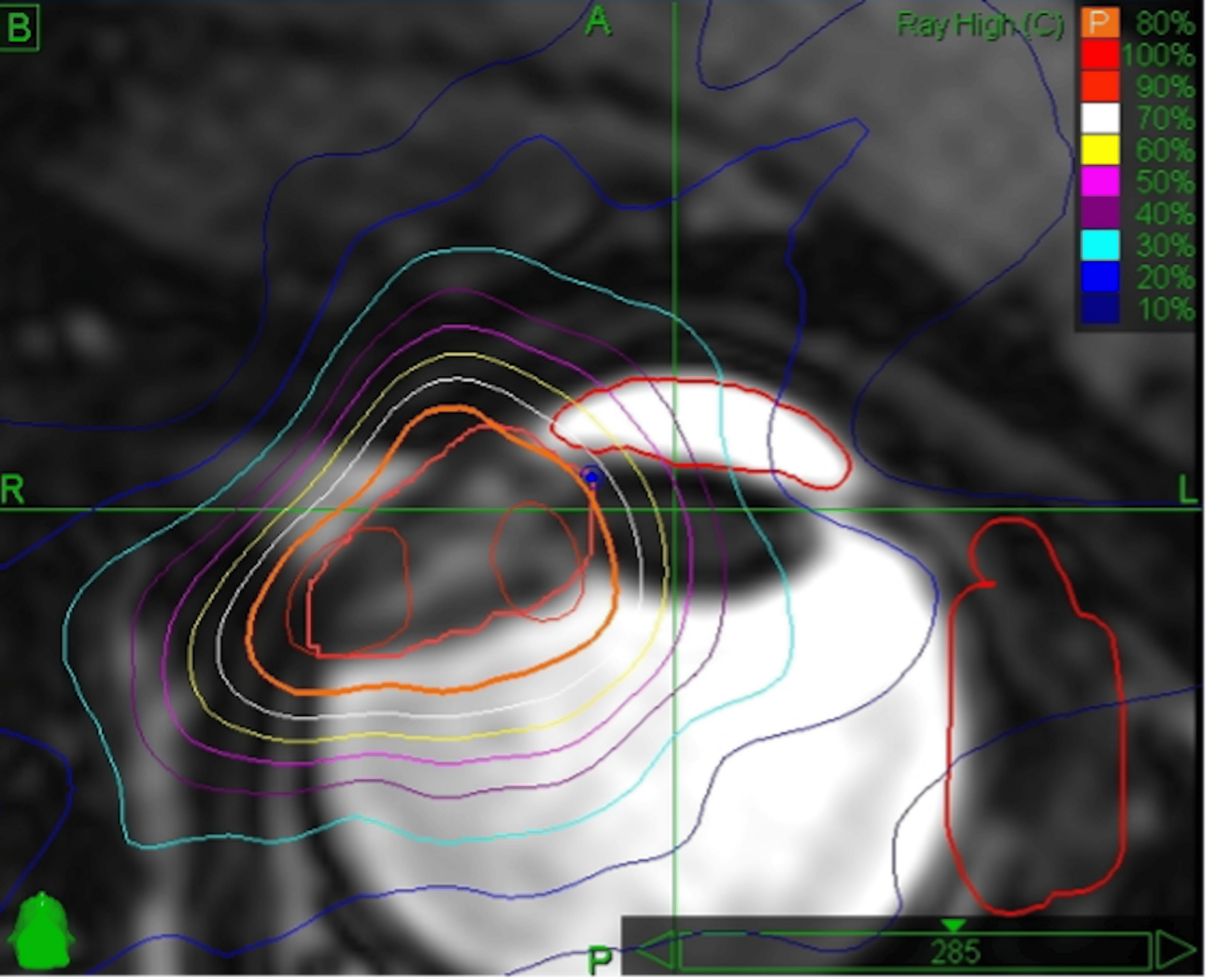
Left eye uveal melanoma with contours traced on CT/MRI fusion; 20 Gy at 80% isodose is prescribed to the PTV (orange) (GTV + 2 mm) with no CTV. PTV: planning target volume; GTV: gross tumor volume; CTV: clinical target volume
